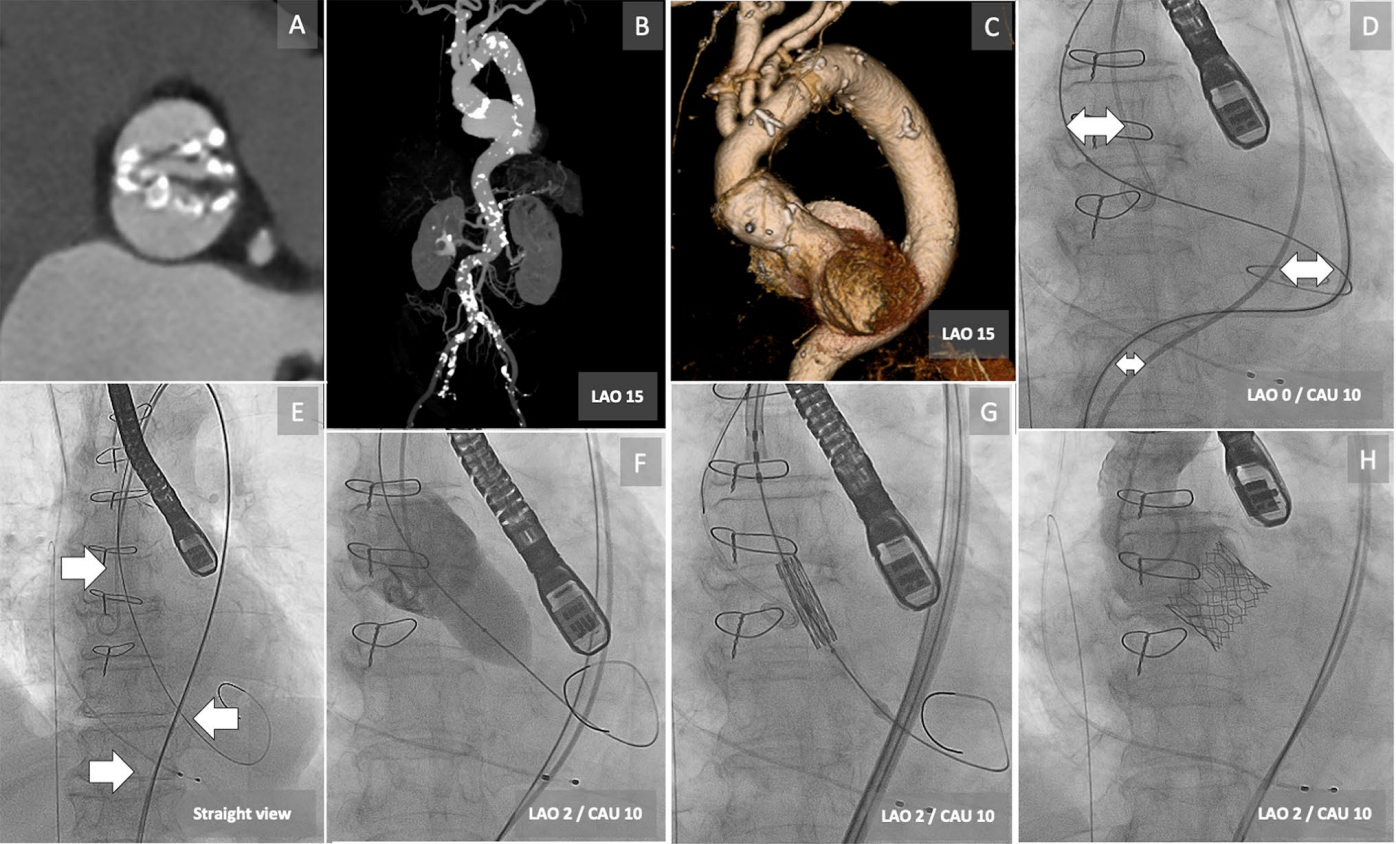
Transfemoral transcatheter aortic valve implantation (TAVI) for bicuspid aortic stenosis with a tortuous aorta after total arch replacement. a 3D computer tomography (CT) reconstruction shows type 0 calcified bicuspid aortic valve. b The tortuous thoracoabdominal aorta. c The synthetic prosthesis of the total arch replacement and the large ascending aorta. d Catheter prolapses (double-headed allows) at the ascending part to the arch graft and the tortuous thoracoabdominal aorta (Online video 1). e Straighten the catheter using double stiff guidewires (allows). f Balloon aortic valvuloplasty with 23-mm balloon. g Easy crossing of SAPIEN 3 through the aorta and aortic valve [Online video 2]. h Good result after TAVI without any complications
